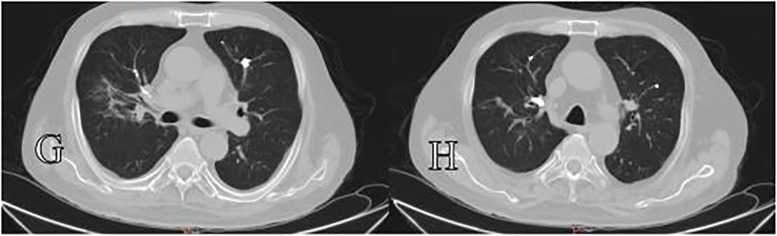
Panels (G,H) show chest computed tomography (CT) scans acquired more than one year postoperatively, which demostrated bone cement emboli within the pulmonary artery branches.
